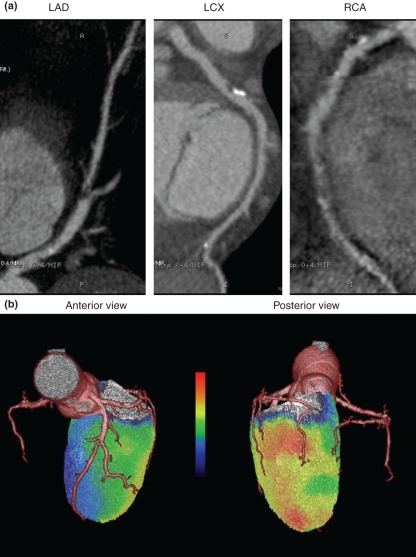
Example of hybrid PET perfusion and CT angiography. a: CT angiography (spiral mode) shows multi-vessel disease with occluded left anterior descending artery (LAD) and poorly visualized mid right coronary artery (RCA). Moderate disease in left circumflex artery LCX. b: Hybrid display during adenosine stress demonstrates severe perfusion reduction in LAD associated region (anterior view) while perfusion was best preserved in LCX related region (posterior view). Perfusion was colour scaled so that red colour denotes 3.5 ml/min/g. Resting perfusion was normal (not shown). Total radiation dose of the hybrid study was 22.3 mSv.
